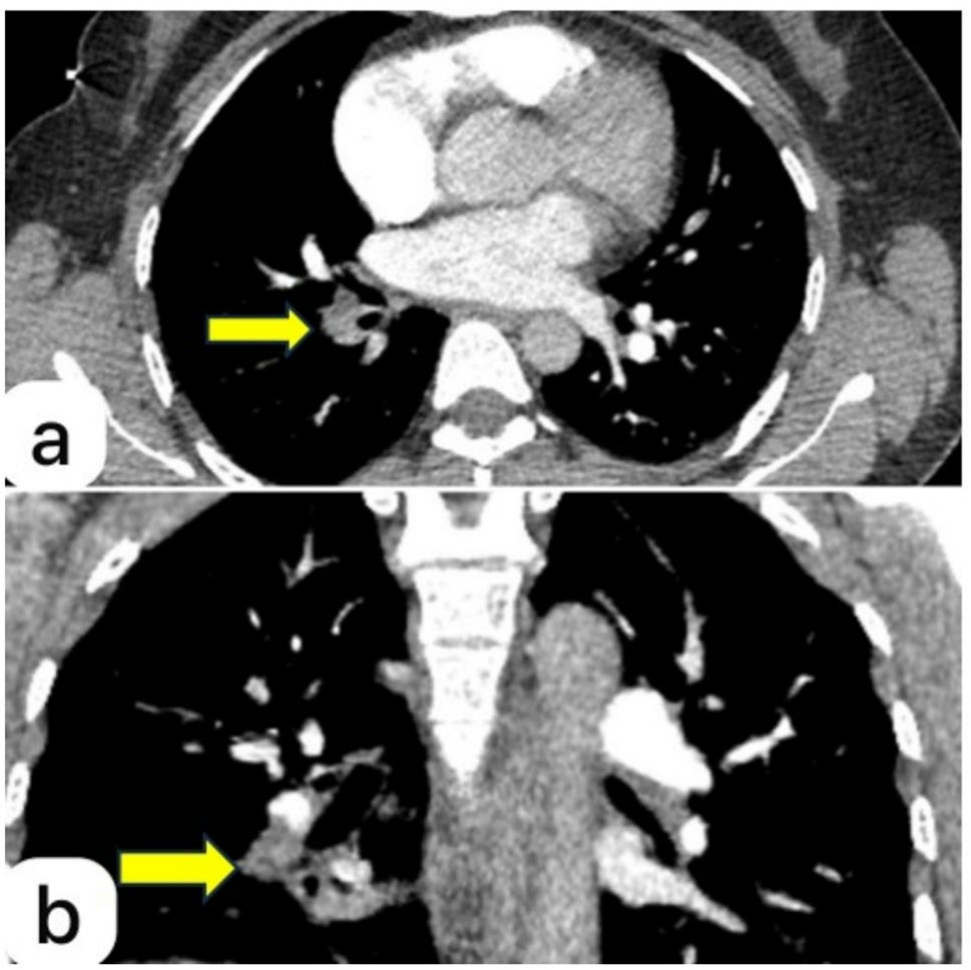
Axial (a) and coronal (b) computed tomography angiography images of the fourth patient showing an obstructive lesion (yellow arrows) in the segmental branch of the right pulmonary artery, consistent with chronic embolic material
